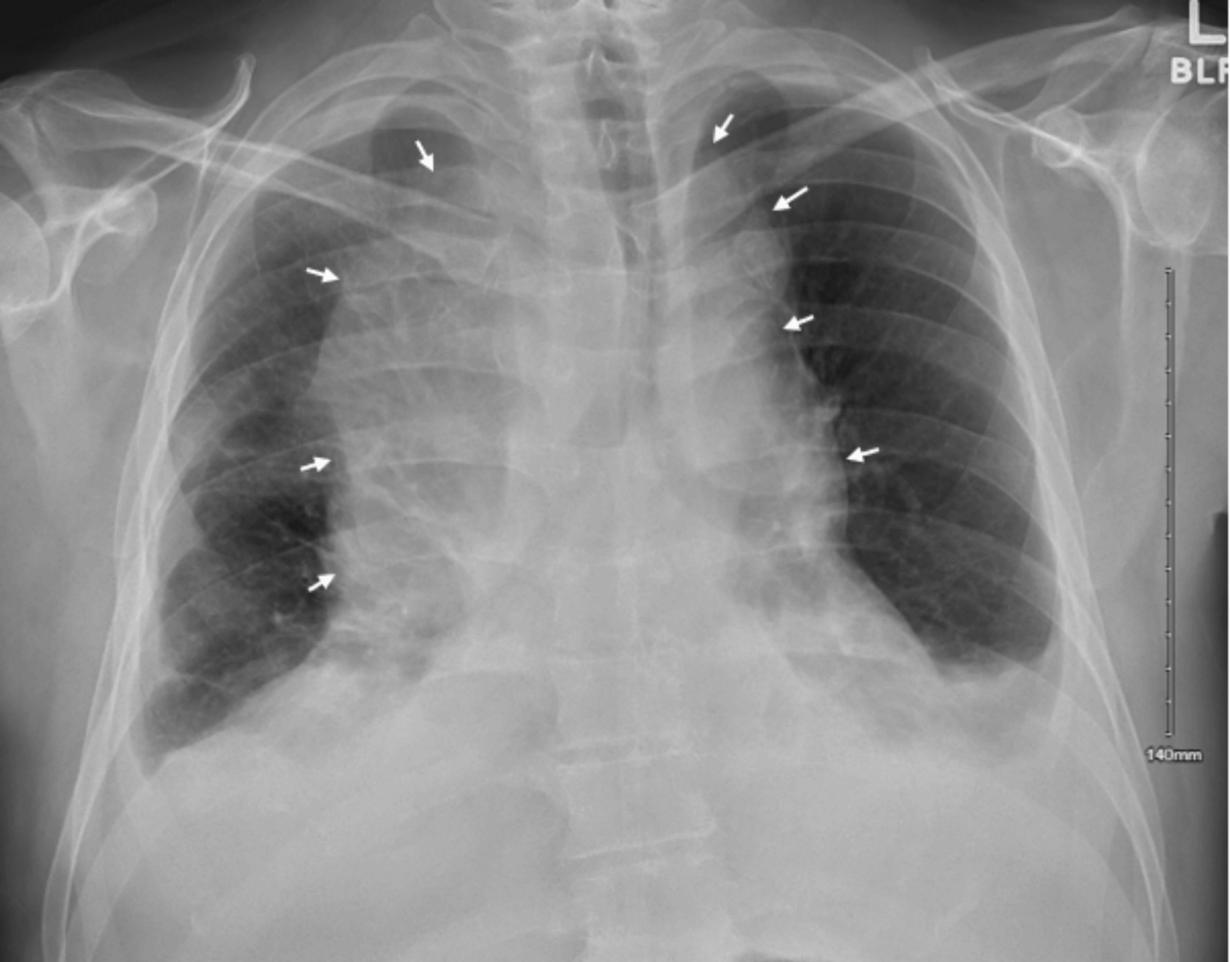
Chest radiograph showing worsening right-sided suprahilar parenchymal density/mass.
